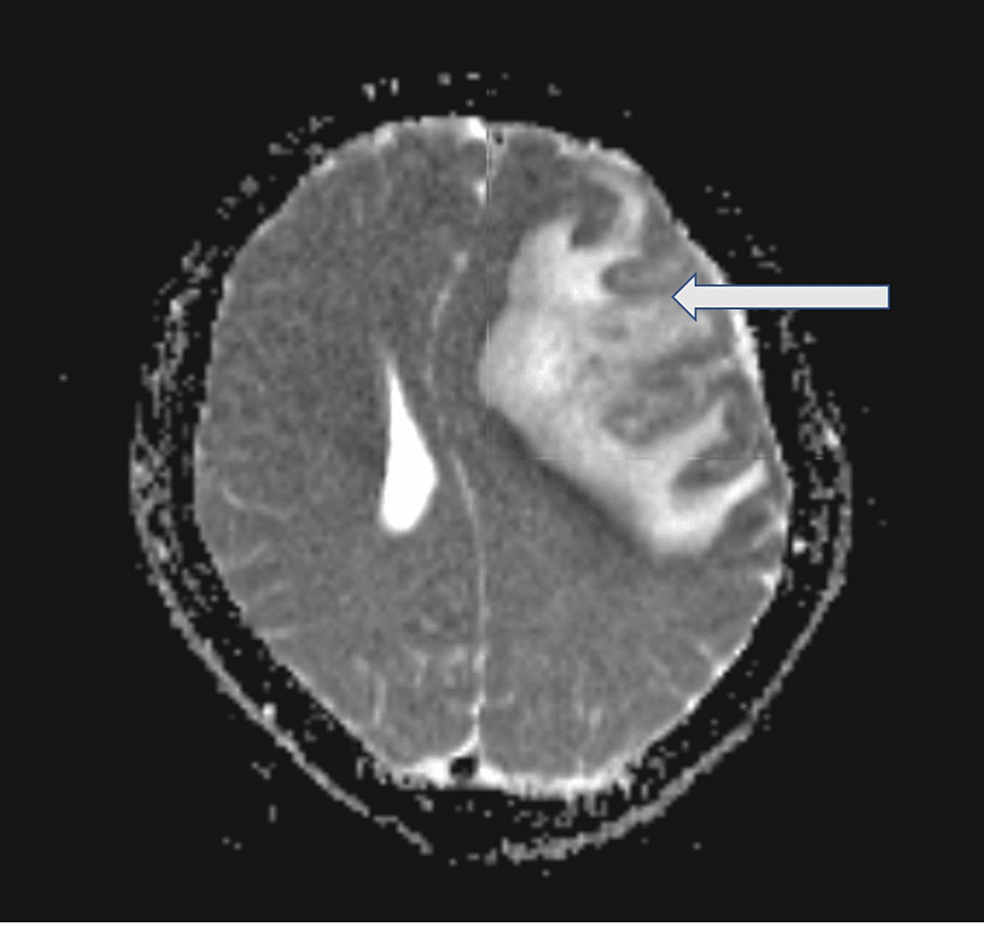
Apparent diffusion coefficient sequence. The magnetic resonance imaging shows an acute left middle cerebral artery infarct.
